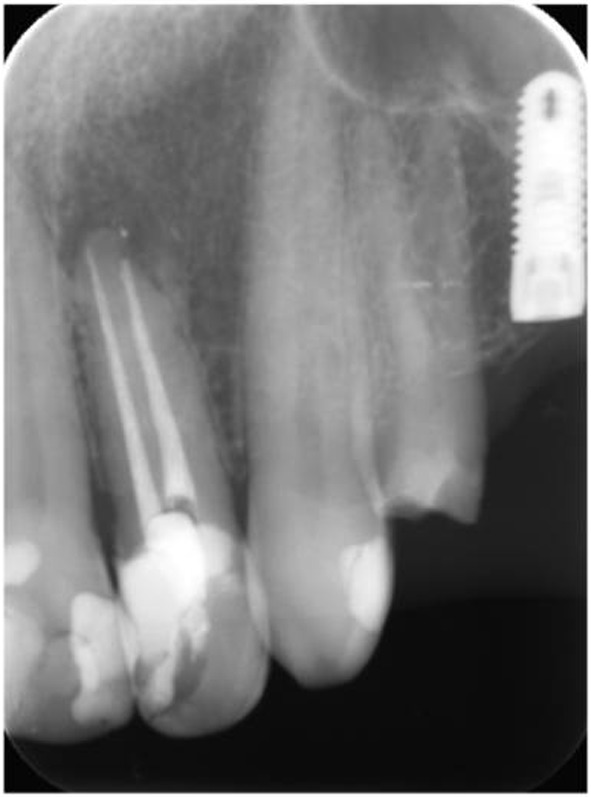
Periapical radiography after 18 months follow-up.
